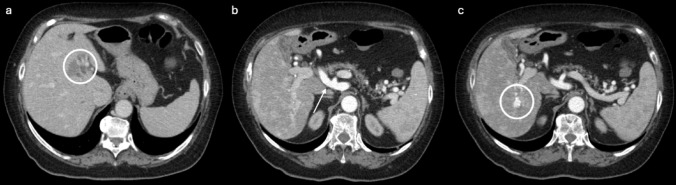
Patient’s CT scans. A Tumor in hilar region suspicious for hilar cholangiocarcinoma with dilation of intrahepatic bile ducts *(white circle)*. B Non-homogeneous parenchymography with multiple spots caused by intrahepatic telangiectasis. Intrahepatic aneurysm between segments 6 and 7 *(white circle)*. C Dilation of hepatic artery *(white arrow)*
